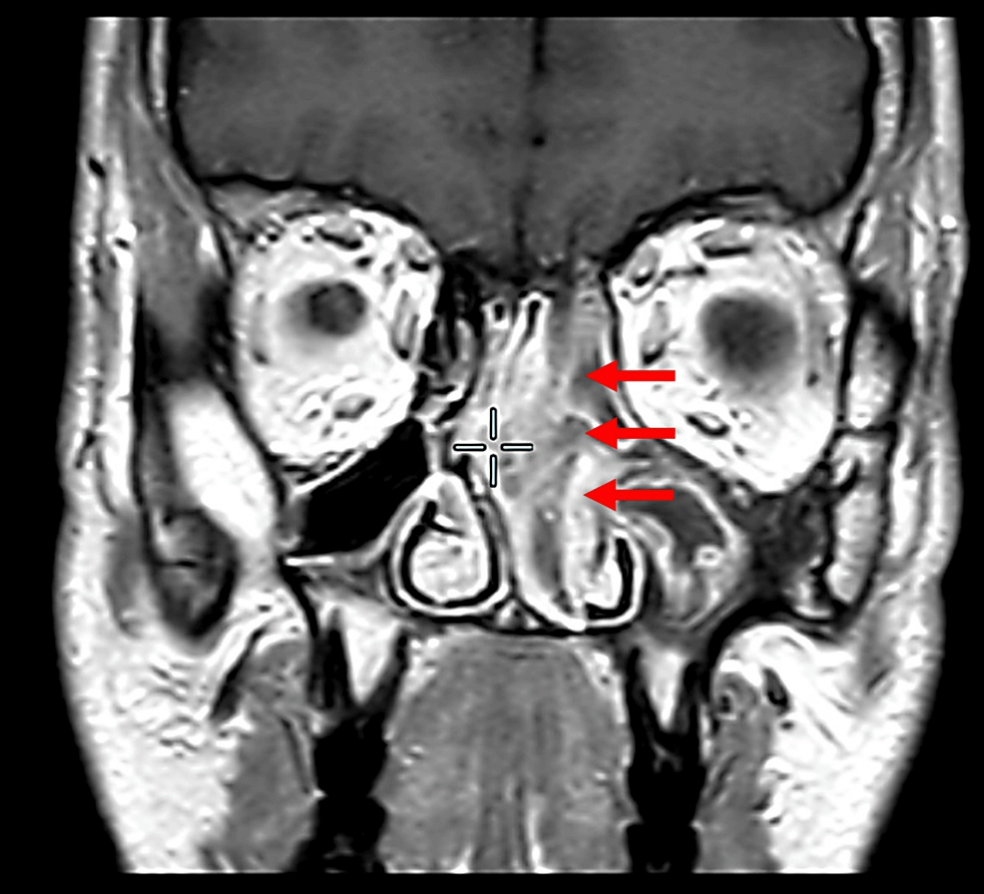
MRI scan (T2 weighted). There was a heterogeneously enhancing lesion noted in the left nasal cavity with a classic cribriform appearance extending into the left maxillary sinus causing ostial widening. The abnormal signal was also extending into the left ethmoid, sphenoid, and frontal sinuses. There was bulging of the medial wall of the left maxillary sinus into the nasal cavity with rightward deflection of the nasal septum. Cribriform appearance seen on the MRI scan (red arrows).
